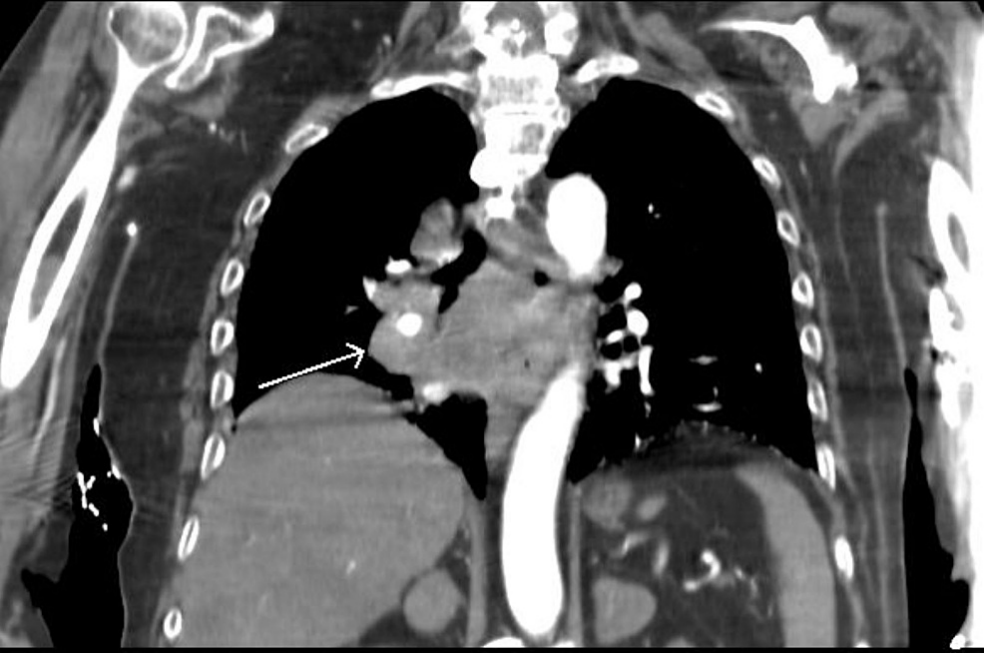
CT of the chest (coronal view) showing a mediastinal mass
